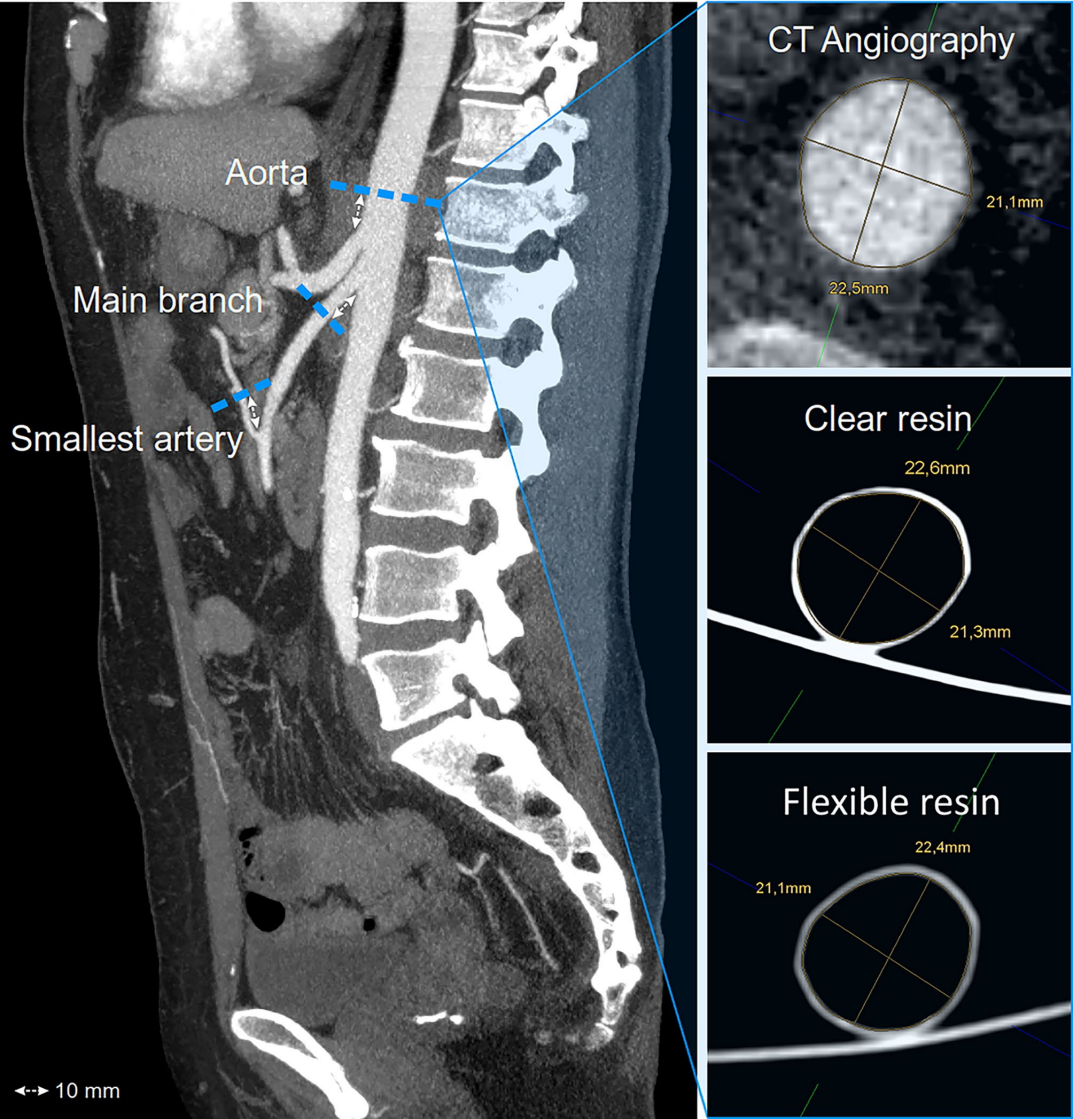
Principle of the diameter measurements of the CT-scanned vascular models and their original CT data. Each case was measured for mean luminal diameters on the patient’s CT scan as well as the related CT-scanned printed models (clear and flexible resin) at three predefined points: (1) the aorta, (2) the procedure-relevant main branch, and (3) the smallest procedure-relevant artery
